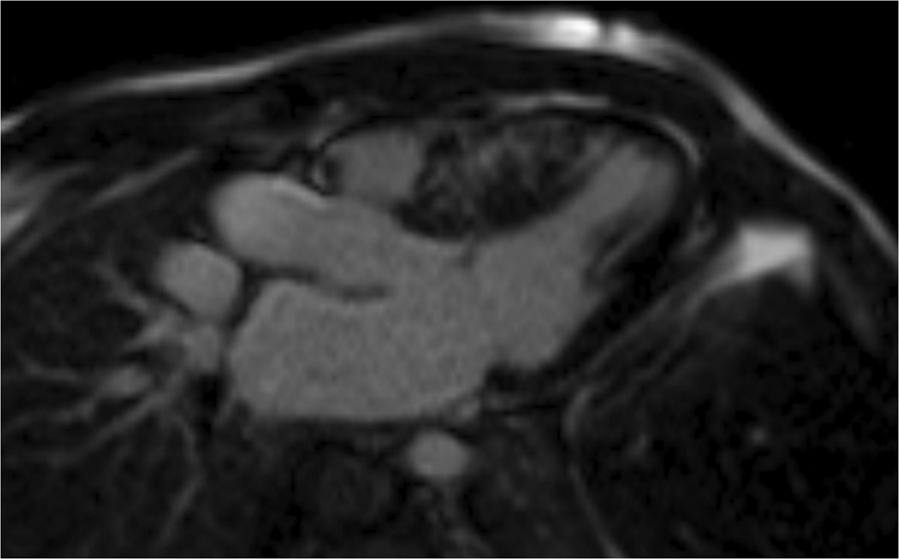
MRI 3 chamber view with delayed gadolinium enhancement. *In the 2 images there is mid-myocardial mural enhancement involving the asymmetrically hypertrophied interventricular septum*
